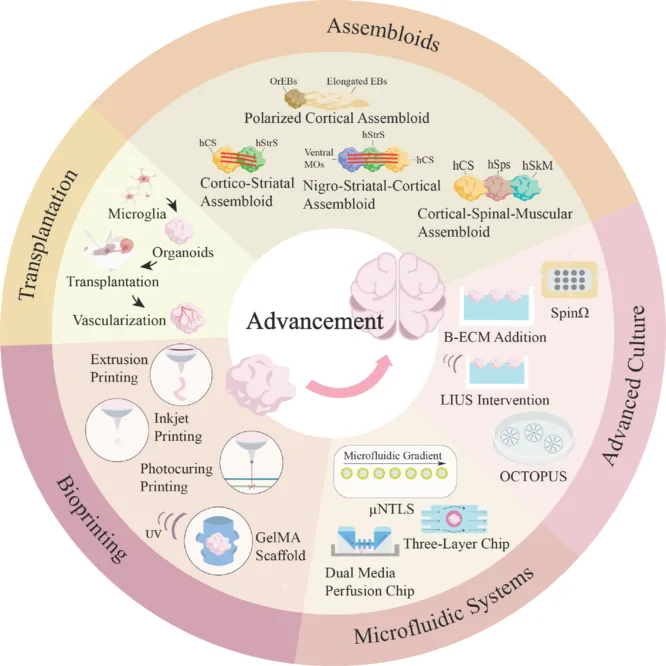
Advancements of organoids. B-ECM: Brain extracellular matrix; EBs: embryoid bodies; GelMA: gelatin methacrylate; hCS: human cortical spheroids; hSkM: human skeletal muscle spheroids; hSpS: human spinal spheroids; hStrS: human striatal spheroids; LIUS: low-intensity ultrasound; MOs: midbrain organoids; OrEBs: organizer-like embryoid bodies; UV: ultraviolet; µNTLS: microfluidic neural tube-like structure.
